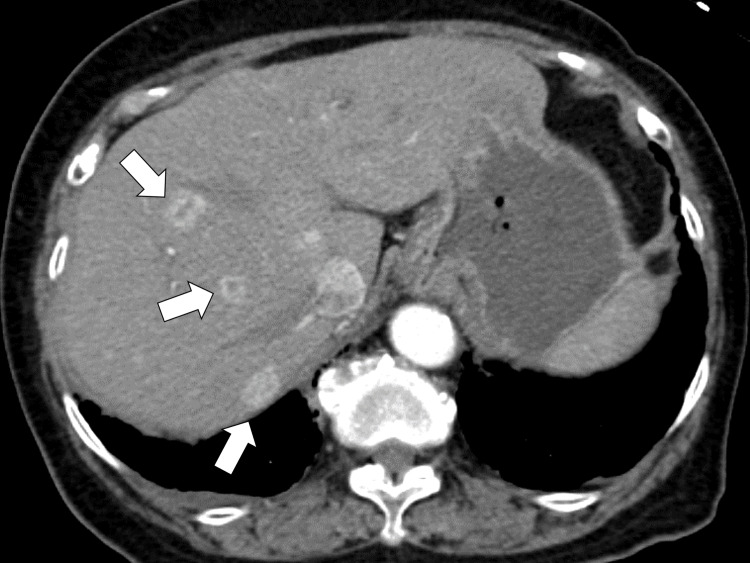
Enhanced chest-to-pelvic computed tomography showing multiple ring-enhanced lesions in the liver (white arrows)
